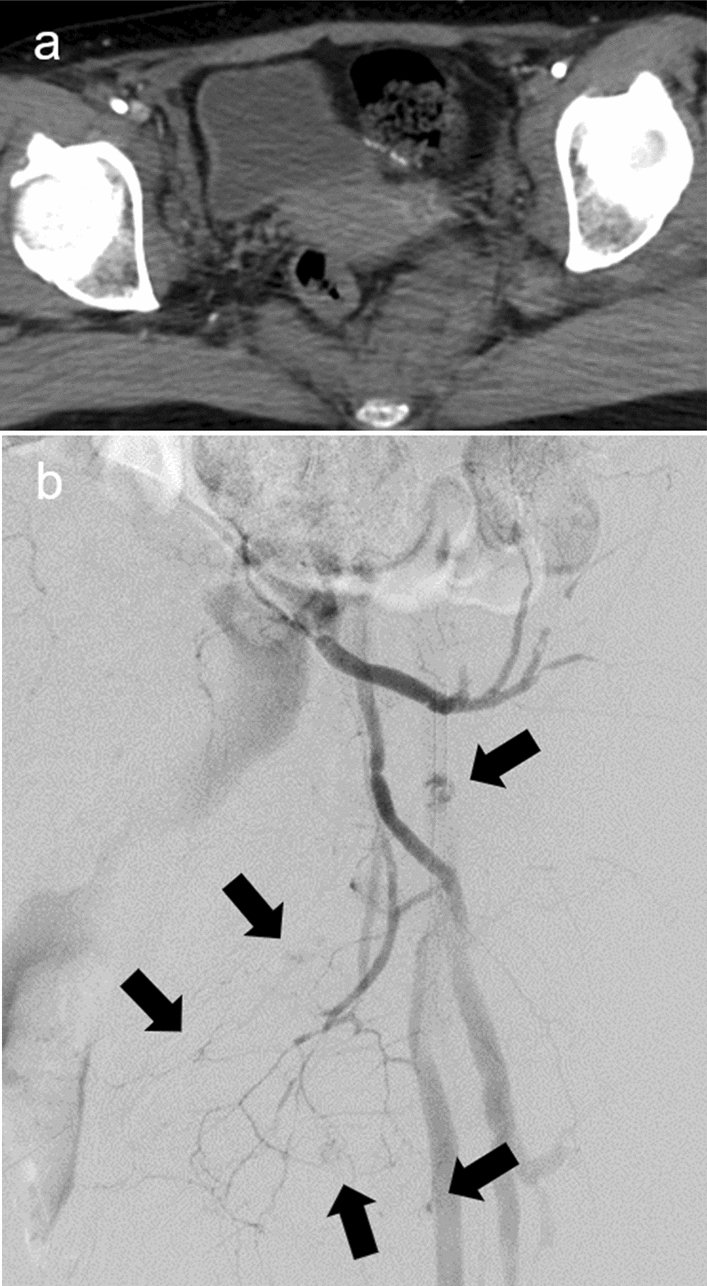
Pelvic contrast-enhancement computed tomography (CT) with complete resuscitative endovascular balloon occlusion of the aorta (REBOA) in Zone I in a patient with pelvic fractures showing a massive hematoma in the retroperitoneal space without extravasation. However, left internal iliac angiography (REBOA was deflated temporarily) shows diffuse extravasation (black arrows), which was hidden by the decreased blood flow under complete REBOA during CT scanning
